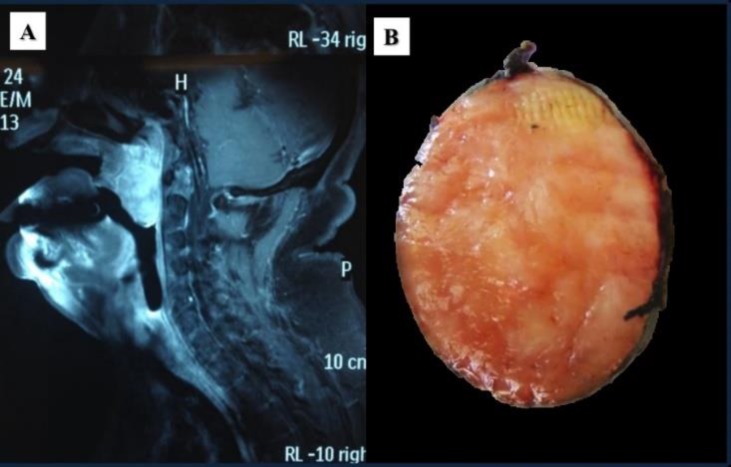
A) Gadolinium-enhanced, sagittal T1-weighted image showing a mass located in the soft palate. B) An ovoid, encapsulated mass measured 3.2x3x2.4 cm. The cut section was firm with a yellow-red surface.
